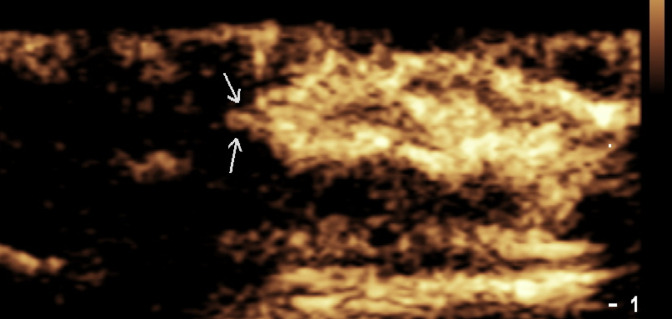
Contrast-enhanced ultrasound image of lower limb lymphatic vessels with minimal contrast agent uptake in a patient with lymphedema; the arrows indicate the lymphatic vessel.
